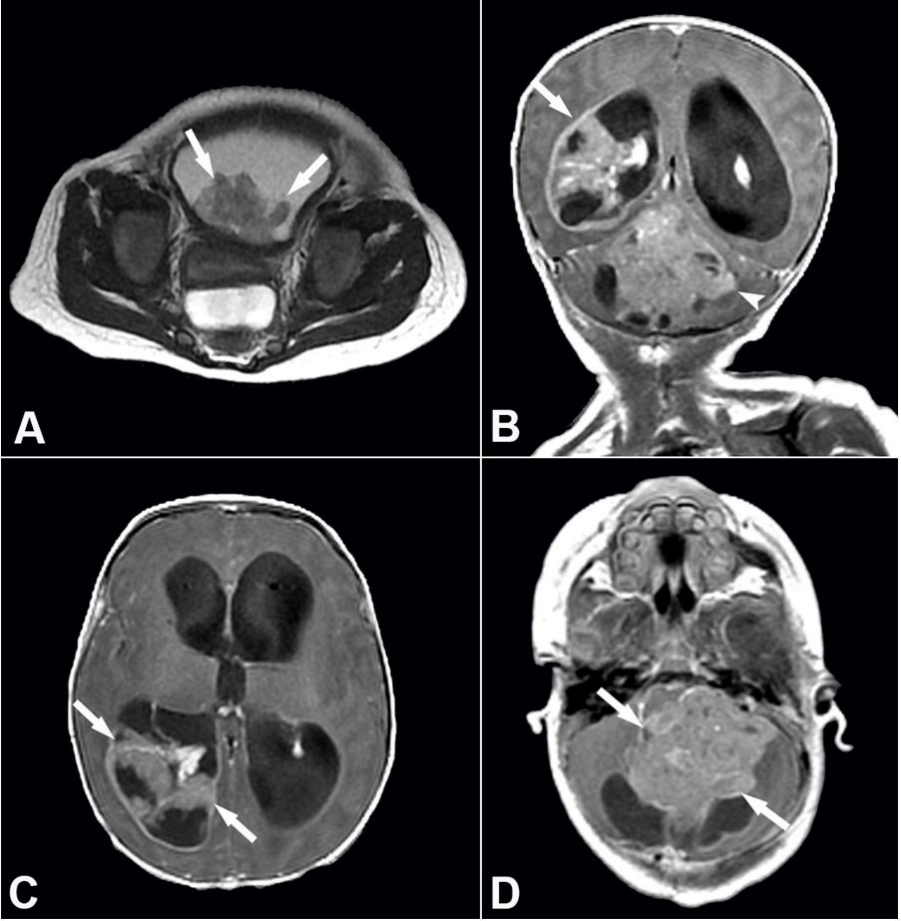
Radiologic imaging of urinary bladder and brain masses. A – Axial T2-weighted image of the pelvis shows a large lobulated mass in the dependent portion of the urinary bladder (arrows); B – Coronal T1-weighted contrast-enhanced image of the brain demonstrates a large heterogeneous mass in the right lateral ventricle (arrow) and the fourth ventricle (arrowhead); Axial T1-weighted contrast-enhanced images of the brain demonstrate a large heterogeneous mass (arrows) in C – the right lateral ventricle and D – the fourth ventricle.
